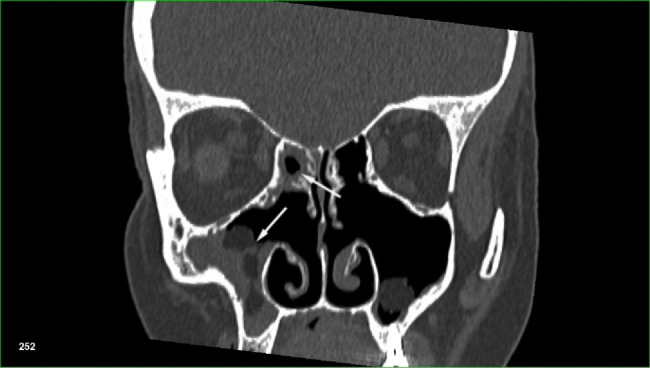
CT scan of the sinuses. Polyposis of the maxillary and ethmoid sinuses (white arrows) in a patient after previous sinus surgery, who underwent allogeneic hematopoietic stem cell transplantation because of primary myelofibrosis.
